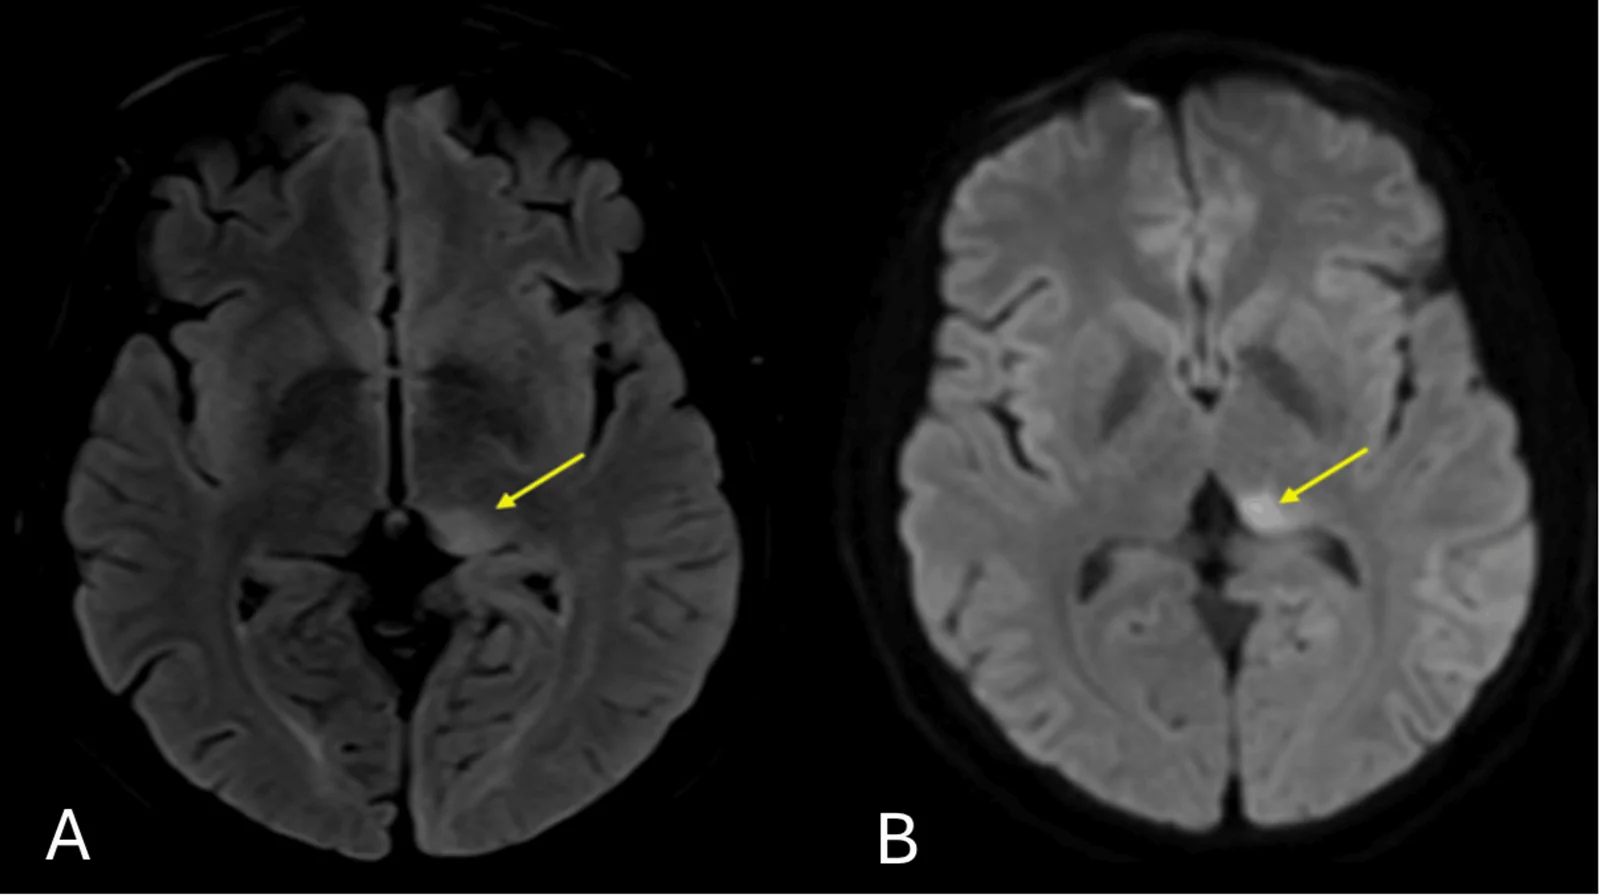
Left thalamic lesion on follow-up MRI FLAIR (A) and DWI (B) MRI sequences demonstrate the development of a new left thalamic lesion. The yellow arrow indicates the left thalamic lesion. MRI: magnetic resonance imaging; FLAIR: fluid-attenuated inversion recovery; DWI: diffusion-weighted imaging
